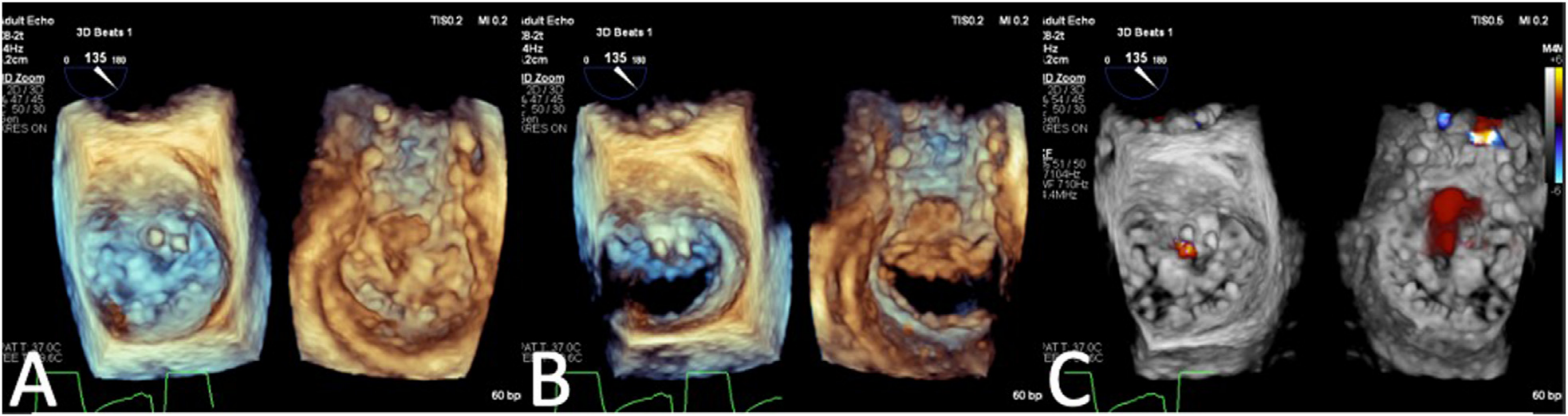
(A) Three-dimensional transesophageal echocardiogram (TEE) shows both plugs safely positioned on the atrial and ventricular aspects of the mitral leaflets. (B) Three-dimensional TEE shows no impairment of mitral leaflet mobility or mitral valve obstruction during diastole. (C) Three-dimensional TEE shows trace residual mitral regurgitation at the end of the case.
